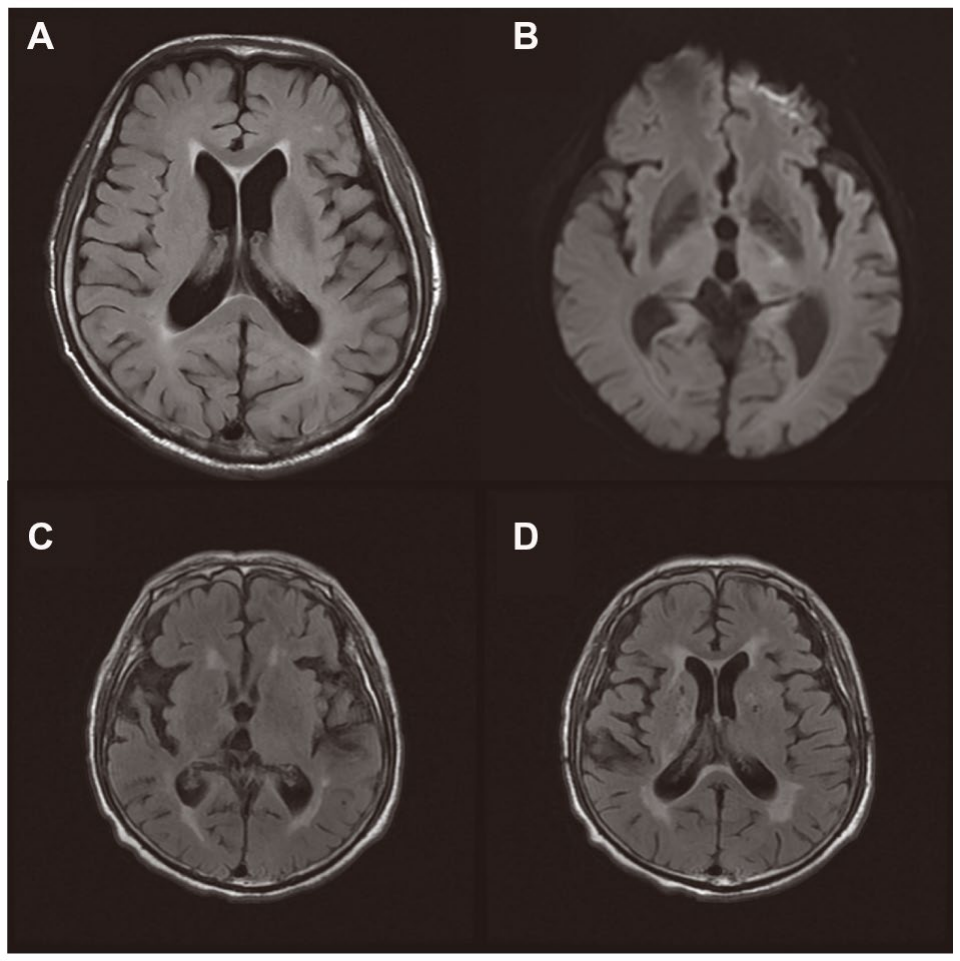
Brain magnetic resonance imaging of two patients during Sepsis-associated encephalopathy (SAE). (A,B): An 75-year-old male patient with serious pneumonia was diagnosed with SAE because of respiratory infection. MRI on day 9 showed high signal on T2-FLAIR sequence, with speckled and patchy WMH in bilateral periventricular cerebral white matter (grade 2 leukoencephalopathy). DWI showed small patchy slightly high signal in the left thalamus region. (C,D): An 79-year-old male patient with urosepsis and cystostomy was diagnosed with SAE. T2-FLAIR sequence showed increased signals in the pontocerebrum, bilateral basal ganglia, radial crown, centrum semiovale, and bilateral frontotemporal parietal lobes.
